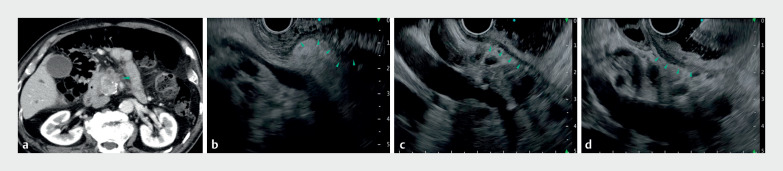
Gel immersion technique in endoscopic ultrasound-guided tissue acquisition (EUS-TA) via the duodenal bulb for a pancreatic head tumor.
